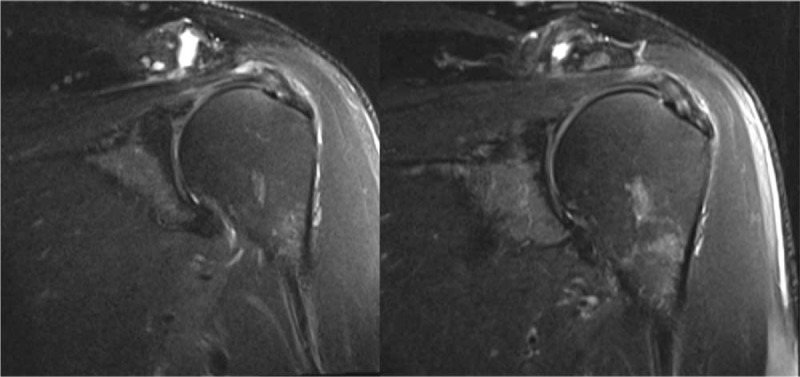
Representative MRI images of rotator cuff pre- and post-injection. Improved signal intensities in the supraspinatus and to a lesser extent the infraspinatus. MRI = magnetic resonance imaging.
